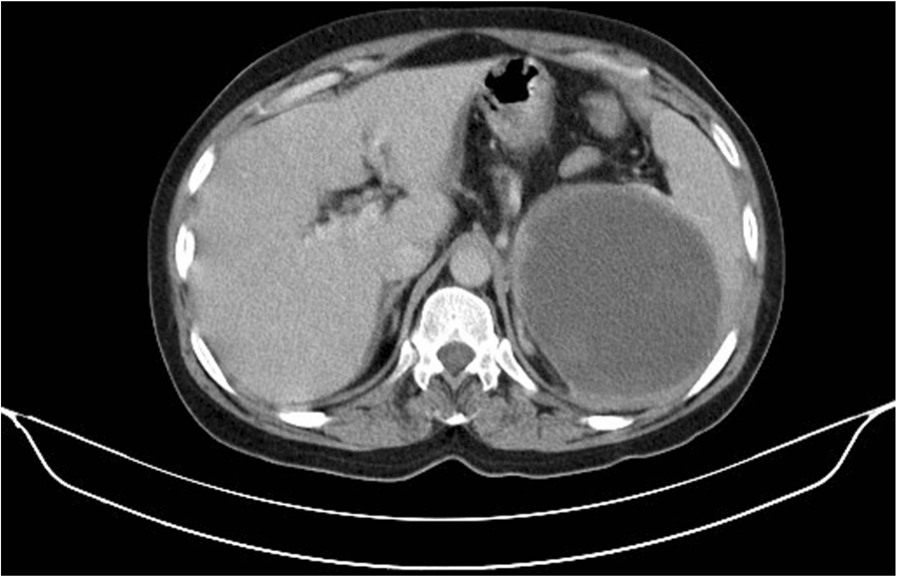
Computed tomography image showing a large hypodense splenic lesion.
